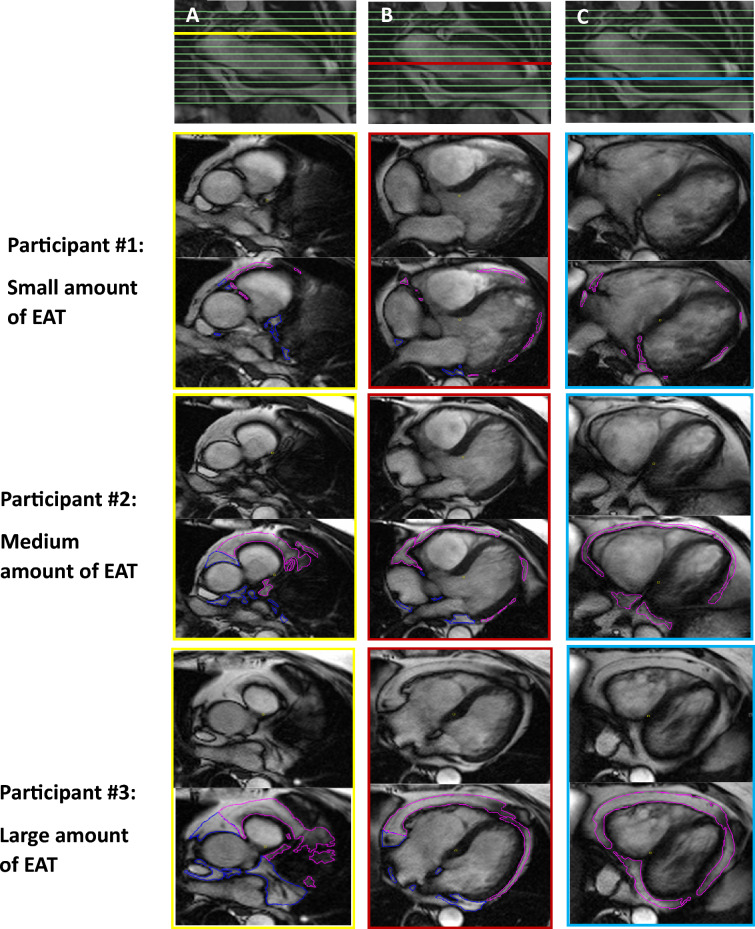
Analyses of cardiac magnetic resonance imaging. Image A–C: To ensure sufficient dimensional orientation, a two-chamber image is used as reference. In this figure, the reference is presented in three different slices marked with yellow, red, and turquoise. Below these images, the axial cine images before and after contouring of the epicardial adipose tissue (EAT) are presented. The volumetric measurements are performed on all slices in a full image stack for all participants, where it is divided into ventricular EAT (pink) and atrial (blue)
